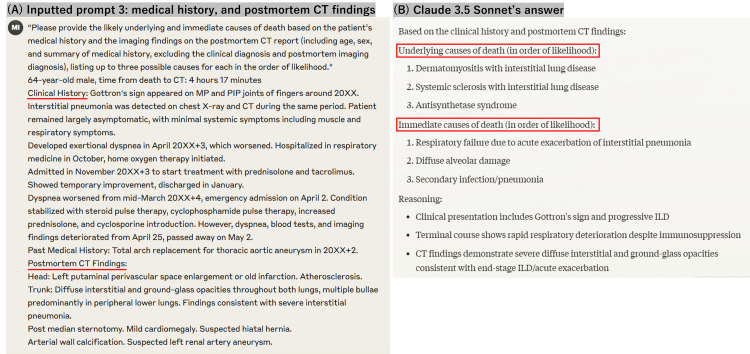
Question for prompt 3 and the response samples (A) Manually typed question for prompt 3, age, sex, time from death to CT, medical history, and postmortem CT findings; (B) Response generated by Claude 3.5 Sonnet
